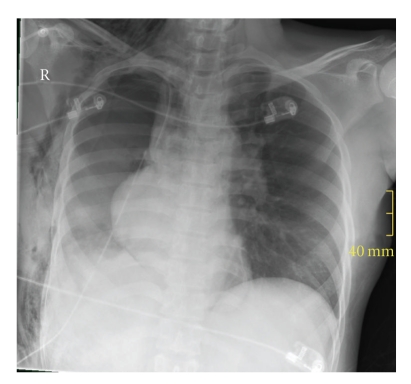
Chest X-rays of the patient with cardiac herniation to the right side.
